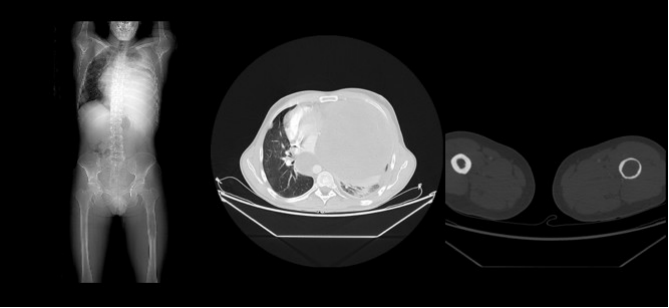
CT Scan showing tumor progression. Eight months after testicular resection, the left lung was completely occupied by a metastatic tumor mass involving mediastinum. The left thigh was also affected by the metastases.
